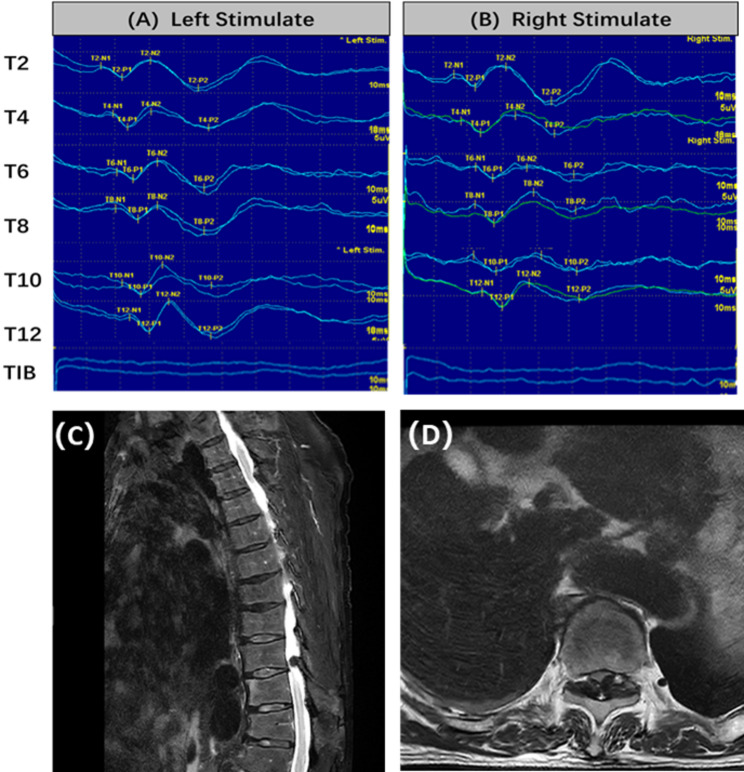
A and B Preoperative electrophysiological recordings obtained during left- and right-sided stimulation in an 81-year-old male patient. Thoracic DSEP waveforms were identifiable from T2 to T12, whereas no reproducible CSEP waveform was observed after medial malleolus stimulation. C and D Sagittal and axial T2-weighted MRI of the thoracic spine, showing spinal cord compression with intramedullary T2 hyperintensity. postoperative neurological improvement was confirmed clinically
